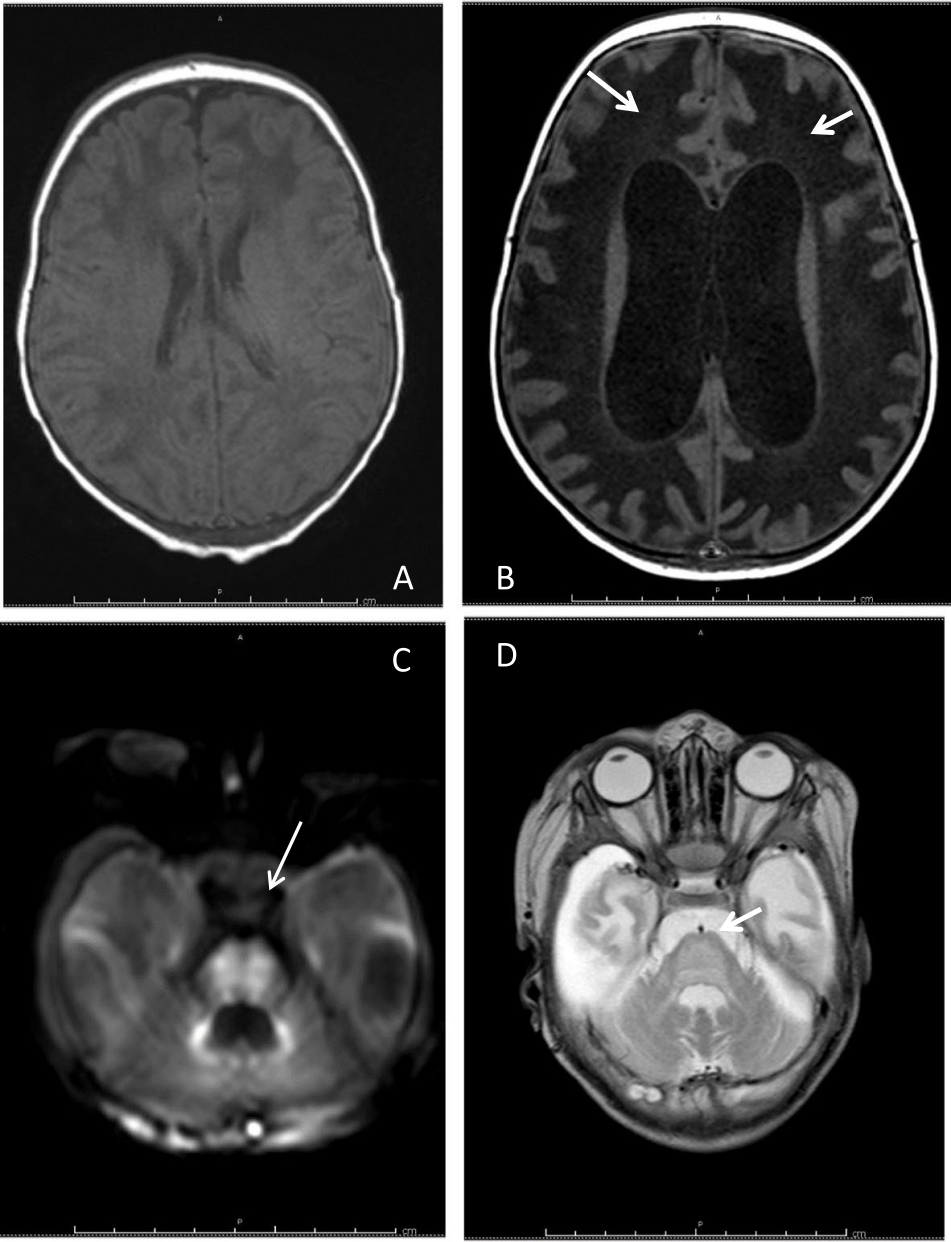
A shows T1 sequences -MRI at three months of life; B represents the T1 sequences- MRI performed at ten months of life. Both images reveal hypointensity of white matter in T1 sequences. C e D were performed before death and showed alteration of the signal and complete involvement of the bulb
